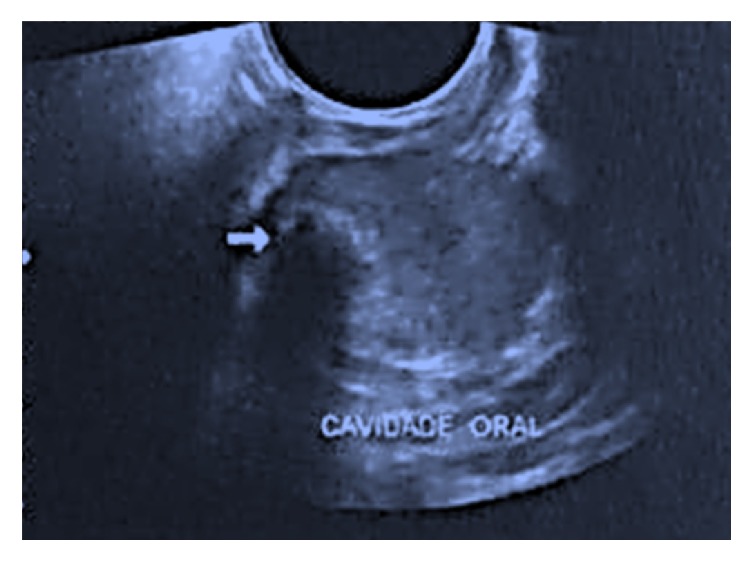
Ultrasonography of the salivary glands. A nodular image of low echogenicity measuring about 2.7 × 1.8 cm was detected.
